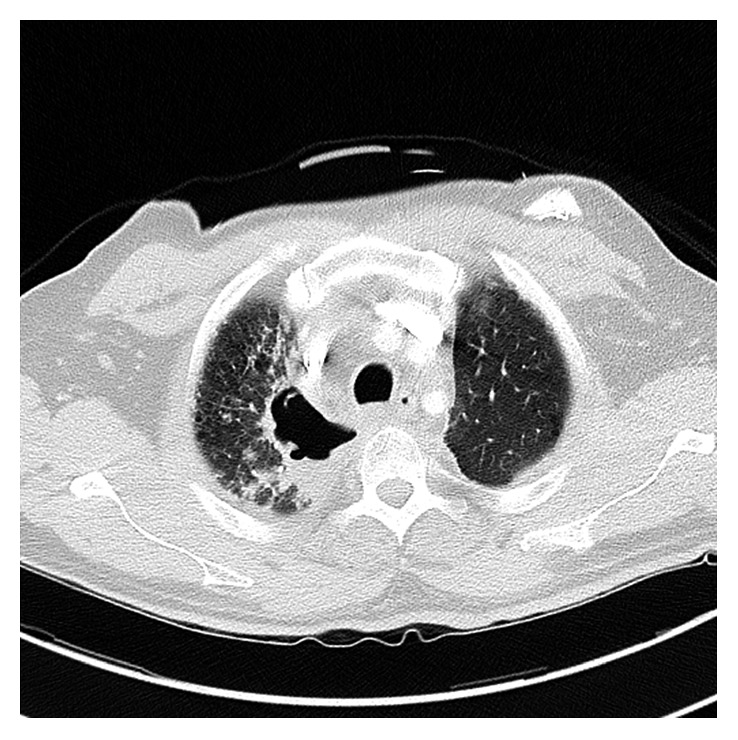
CT chest showing air-fluid levels within chest after initiation of chemoradiation.
